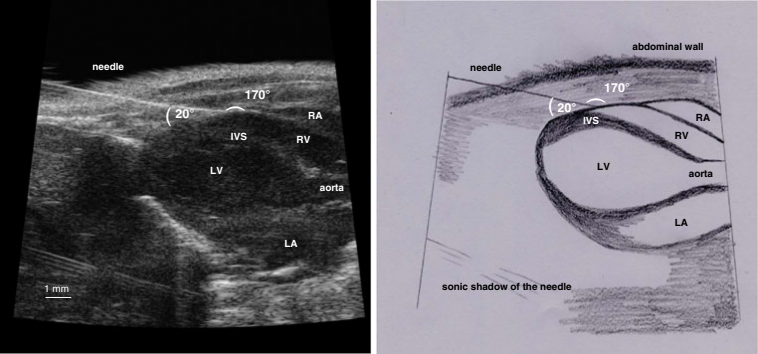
Echocardiographic visualisation of pericardial injection: modified echocardiographic LAV showing the position of the needle tip inside the pericardial sac for the pericardial injection in characteristic RV position (*left*) and scheme (*right*). *IVS* interventricular septum, *LA* left atrium, *LV* left ventricle, *RA* right atrium, *RV* right ventricle
